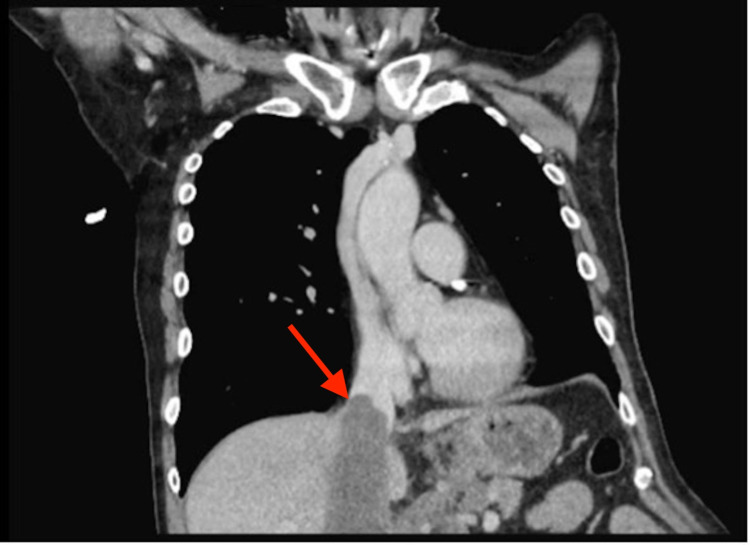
CT scan with frontal view of inferior vena cava thrombus
